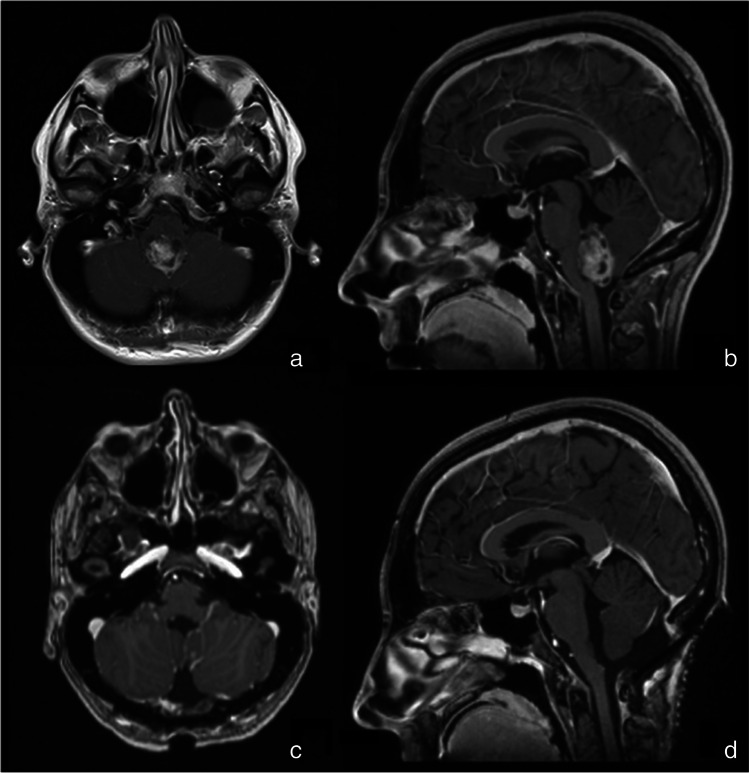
Preoperative (a and b) and postoperative (c and d) images of patient number 6 who underwent SF guided surgery. T1-weighted image with gadolinium shows complete resection of the lesion
